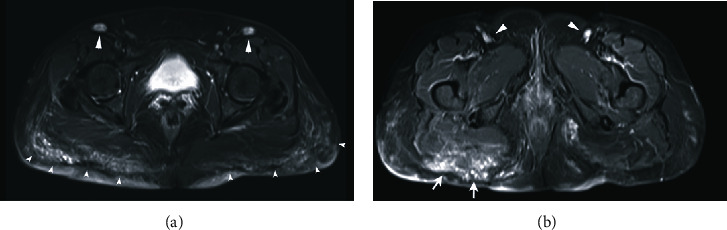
MRI using STIR sequence in the axial plane shows infiltration of the ganglia and the different patterns present in the gluteal region. Unknown substance, 2000 ml. (a) Depicts a mixed globular and diffuse pattern that affects the gluteus maximus muscle and the subcutaneous cellular tissue of the gluteal regions (small and large, white arrowheads) migration to the left ischiorectal fossa. (b) Infiltration by modelling material of the inguinal ganglia and gluteus maximus muscle (white arrows and arrowheads). Infiltration of ganglia points to the engulfment of injectable filler by macrophages and their eventual migration to regional ganglia.
